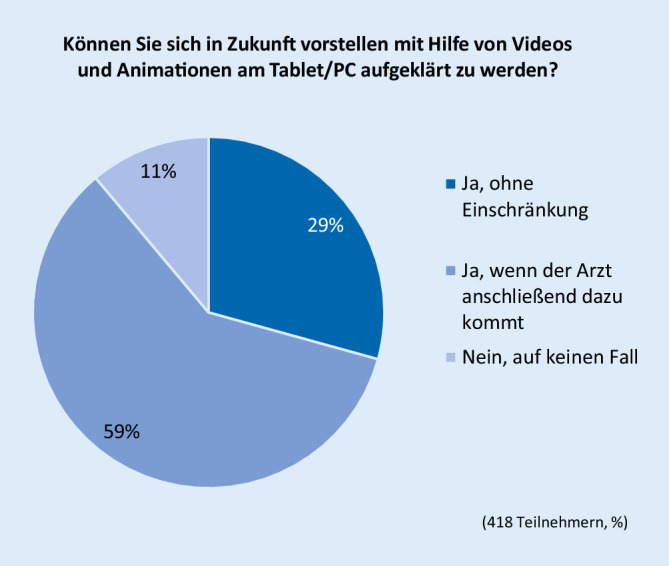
### Befragung unter Ärzten zu Aufwand und Verbesserungsmöglichkeit bei der Aufklärung für CT-Untersuchungen
